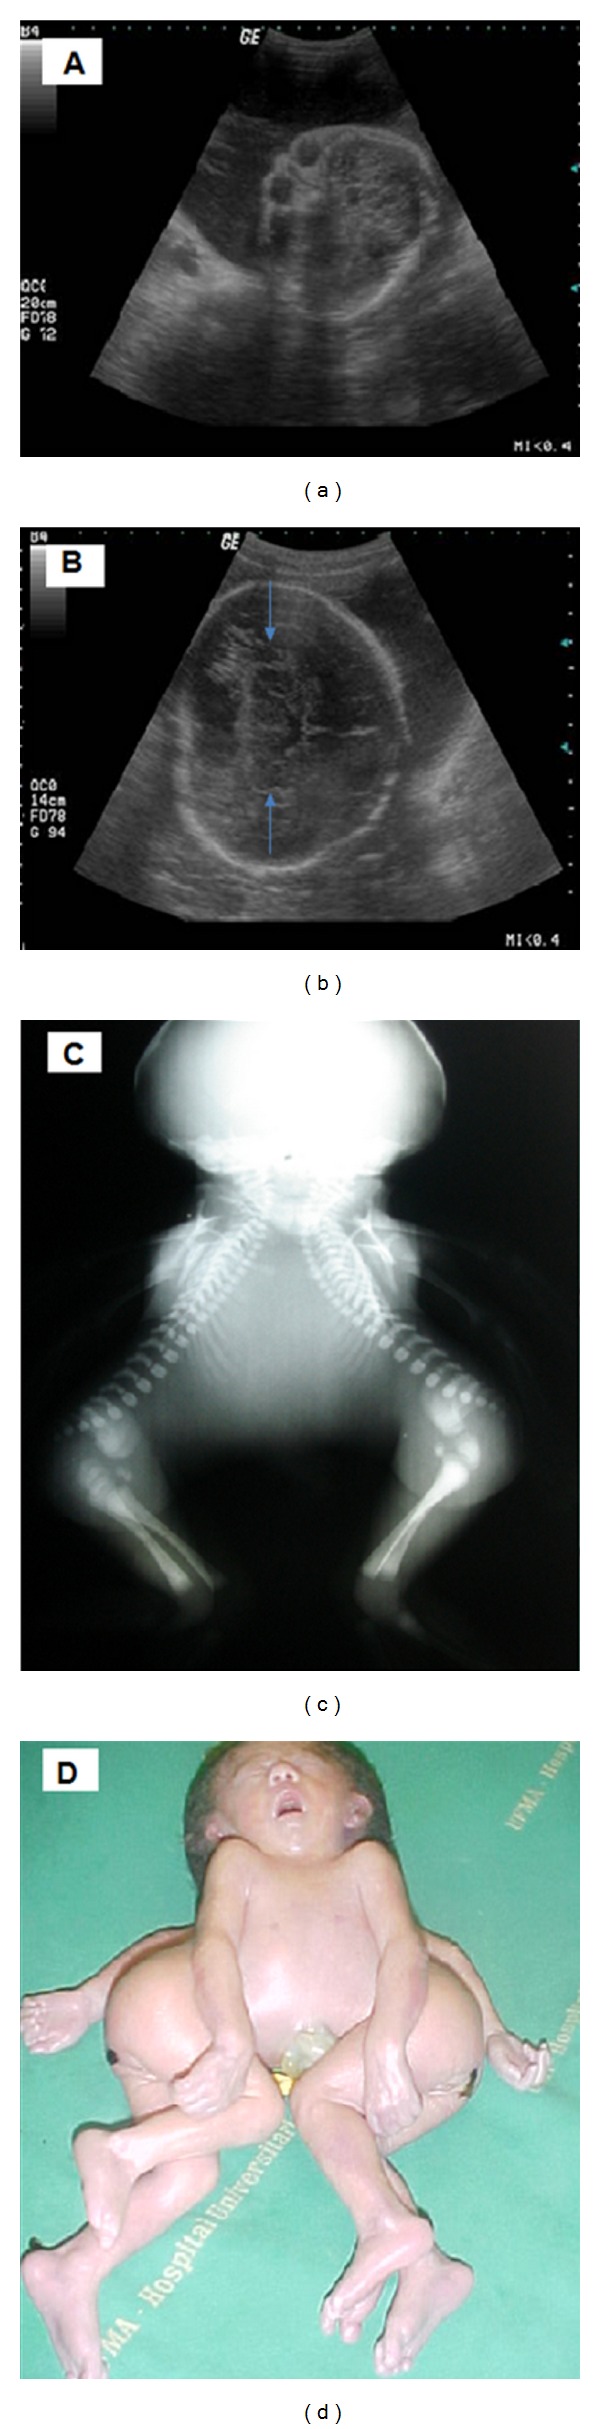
Cephalothoracopagus janiceps disymmetros. (a) Prenatal two-dimensional ultrasound showing only a skull with two faces and two eyes. (b) Prenatal two-dimensional ultrasound showing the brain with the thalamus fusion (blue arrows). (c) Postnatal X-ray showing the fusion of twins from head until upper abdomen. (d) Postnatal *postmortem* analysis confirming the prenatal diagnosis.
